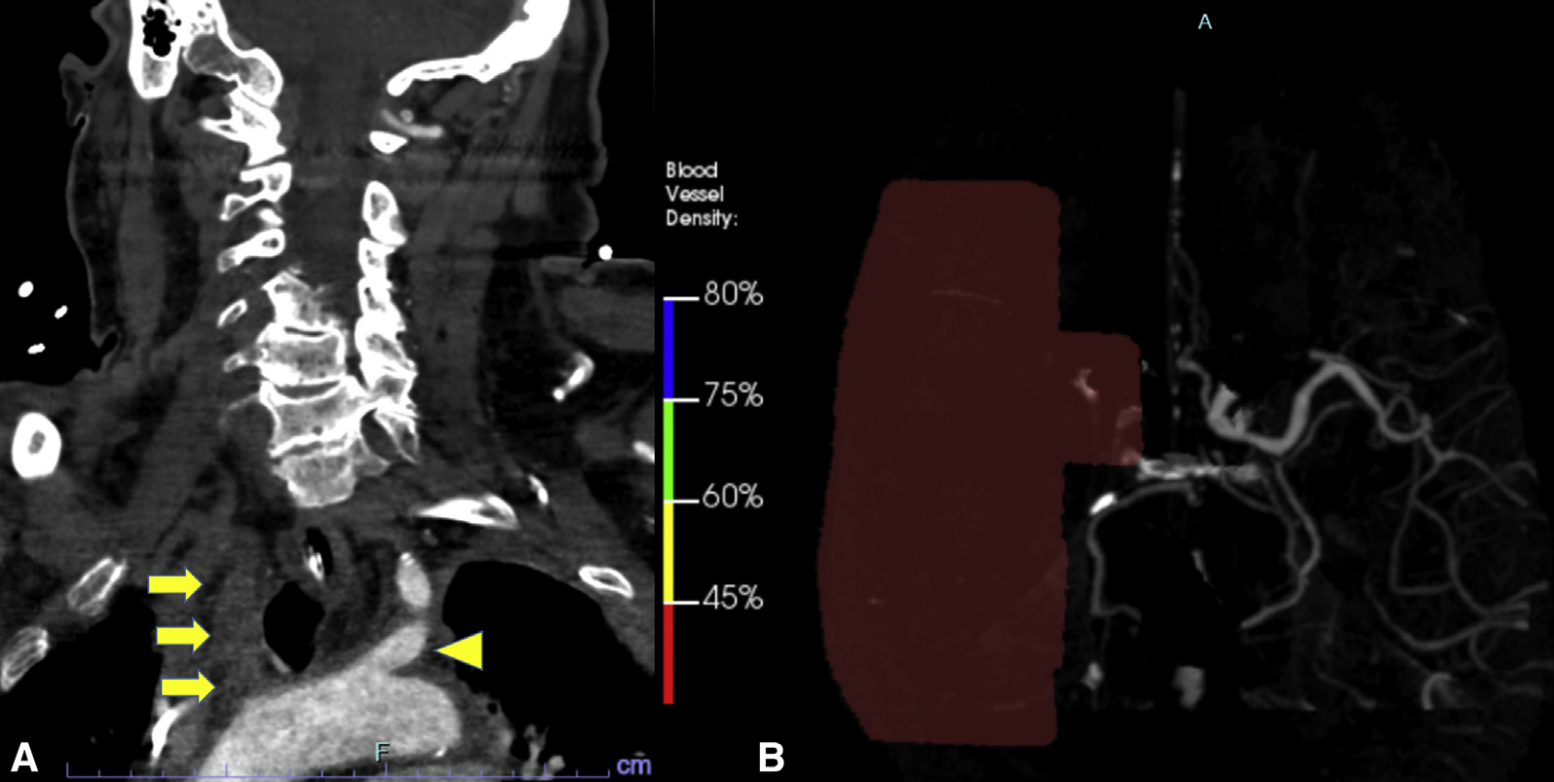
A male patient required venoarterial extracorporeal membrane oxygenation (VA-ECMO) via the right axillary artery due to postcardiotomy shock after ischemic ventricular septal defect repair. On postoperative day 2, with recovery of left ventricular function, the patient suddenly developed left hemiparesis with a dropped VA-ECMO flow. Emergent computed tomography (A) showed occlusion of the innominate artery (*arrows*) to the right common carotid artery. A brain perfusion scan showed a complete occlusion of the right middle cerebral artery system (*red area* in B). An *arrowhead* shows the left subclavian artery.
